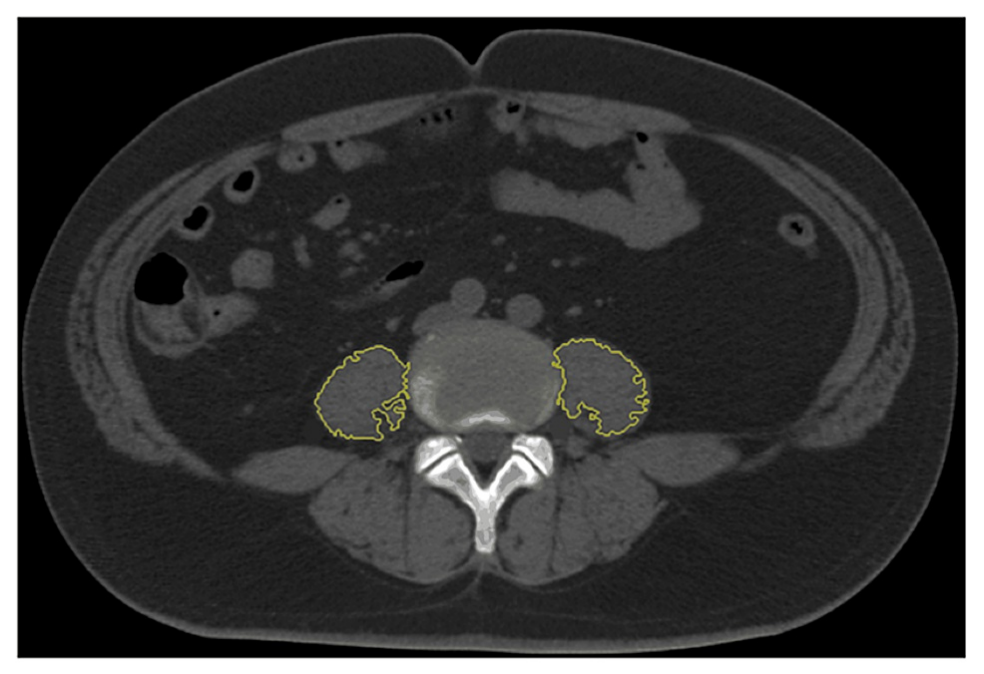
Measurement of psoas muscle area on CT Measurement of psoas muscle area at the third lumbar spine level on CT. The psoas muscle borders were manually outlined (manual trace method). Each PMI was calculated using right and left areas enclosed in line.
